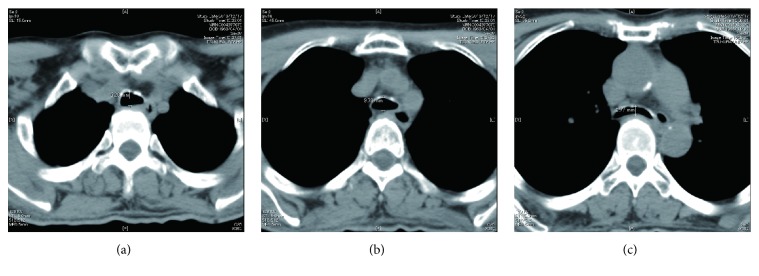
Chest computed tomography 1.5 months previously. (a) The anteroposterior diameter of the trachea at 3 cm away from the glottis was 10.3 mm. (b) The anteroposterior diameter of the trachea at 6 cm away from the glottis was 9.8 mm. (c) The anteroposterior diameter of the tracheal bifurcation at 9 cm away from the glottis was 5.8 mm.
